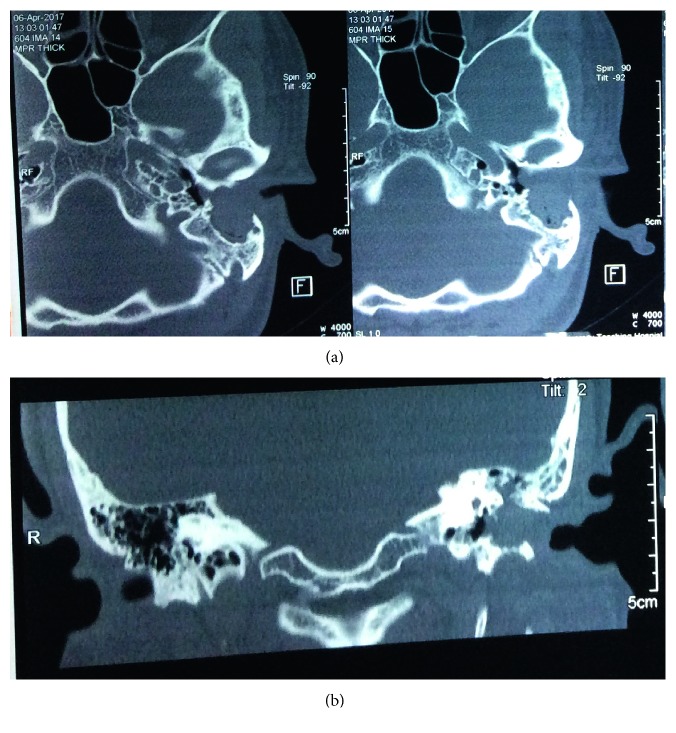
(a) High-resolution computed tomography (HRCT) temporal bone axial view showing homogeneous soft-tissue density filling the left EAC extending into the mastoid cavity destroying the posterior wall of EAC, focal destruction of anterior canal wall. Medial bowing of the tympanic membrane can also be seen. (b) High-resolution computed tomography (HRCT) temporal bone coronal view showing homogeneous soft-tissue density filling the left EAC extending superiorly and inferiorly with focal bony destruction.
